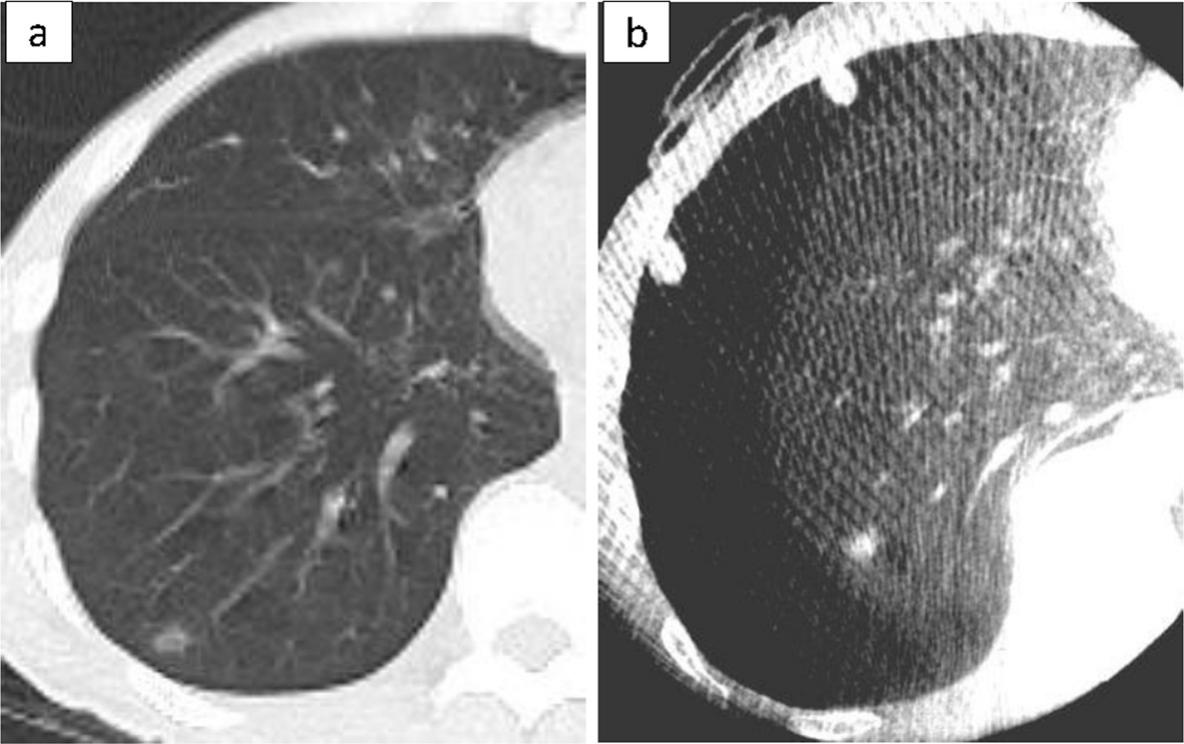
A GGN without a solid part. Preoperative CT shows a GGN, 9 mm in diameter, without a solid part (a). When the lung is incompletely re-expanded, the O-arm shows a higher density lesion than the preoperative CT finding, similar to a solid tumor (b).
